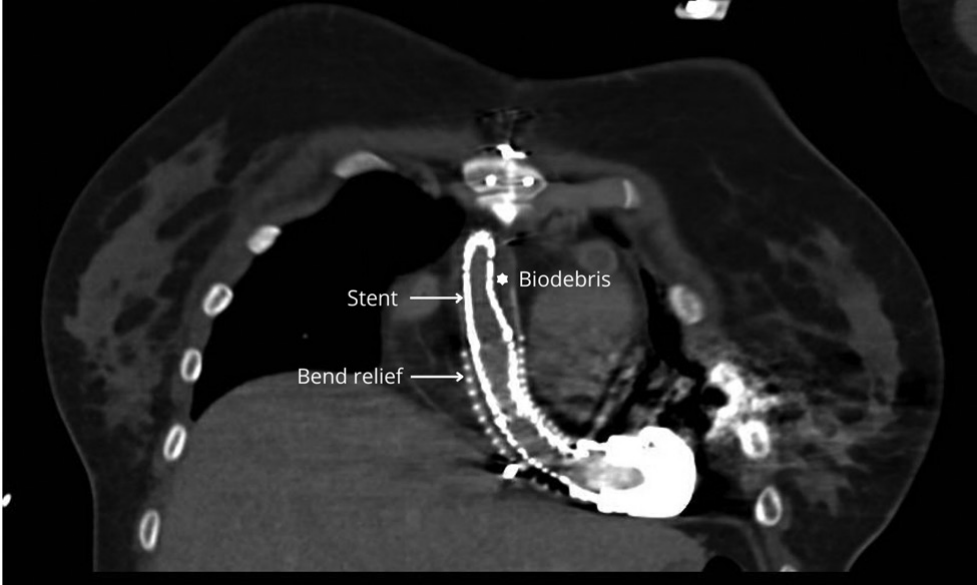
CTA scan 1 year after stenting of the OG, showing good proximal expansion of the stented segment. Mild tapering is seen at the cranial (distal) portion of the stent, near the aortic anastomosis, likely due to migrated biodebris during the initial procedure, resulting in residual external compression. CTA, computed tomography angiography; OG, outflow graft.
